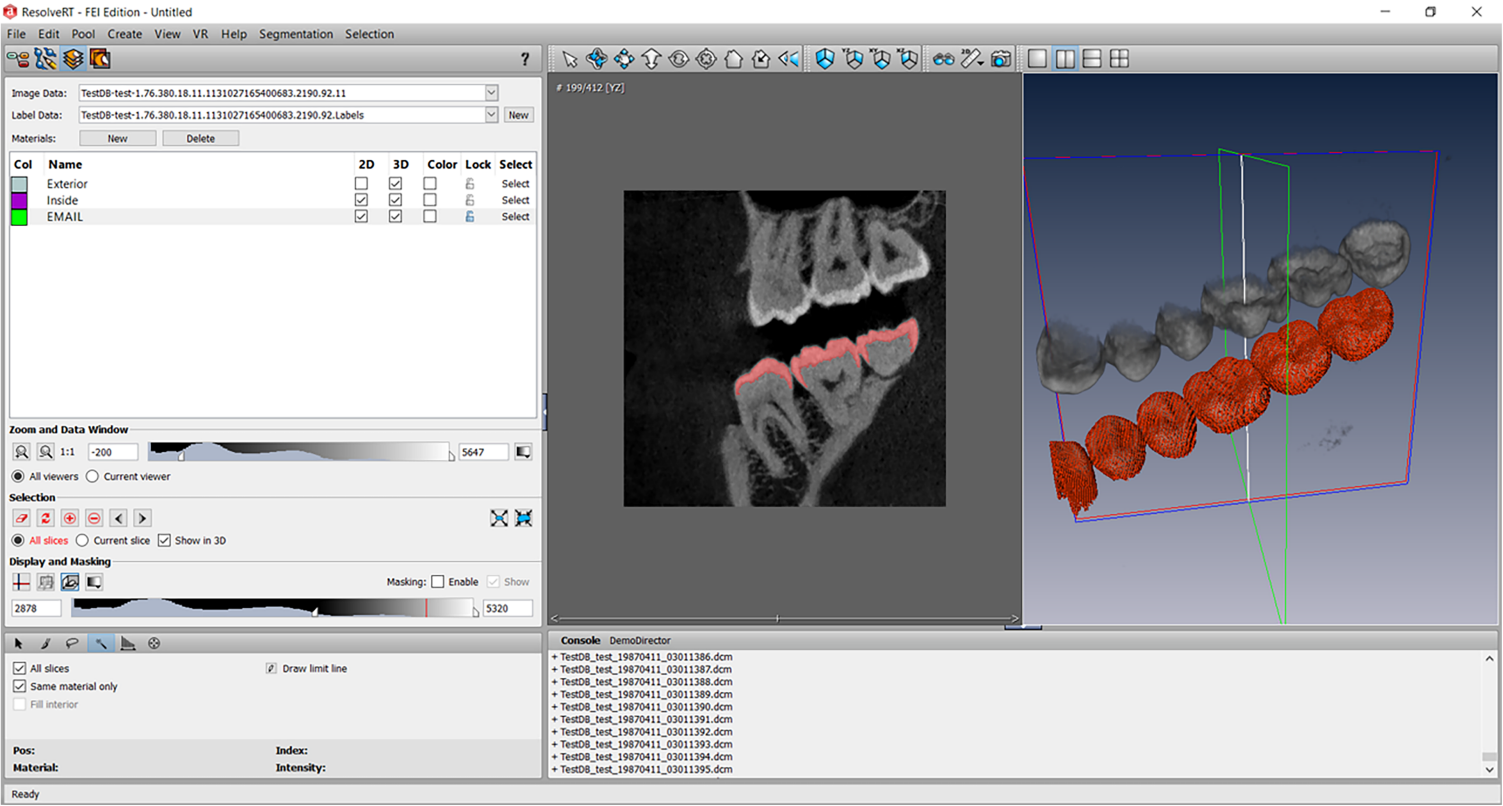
Screenshot of the Amira software segmentation of enamel on all views of the CBCT acquisition according to the gray level of the structures. Other structures were segmented using the same method.
